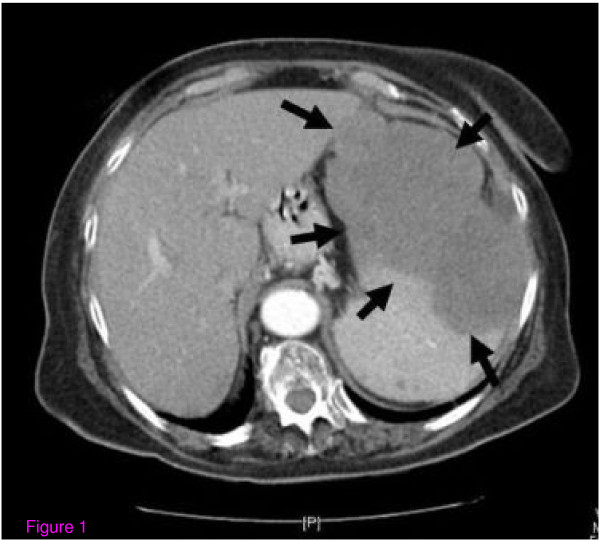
An abdominal CT scan of the patient during the first hospital admission.
